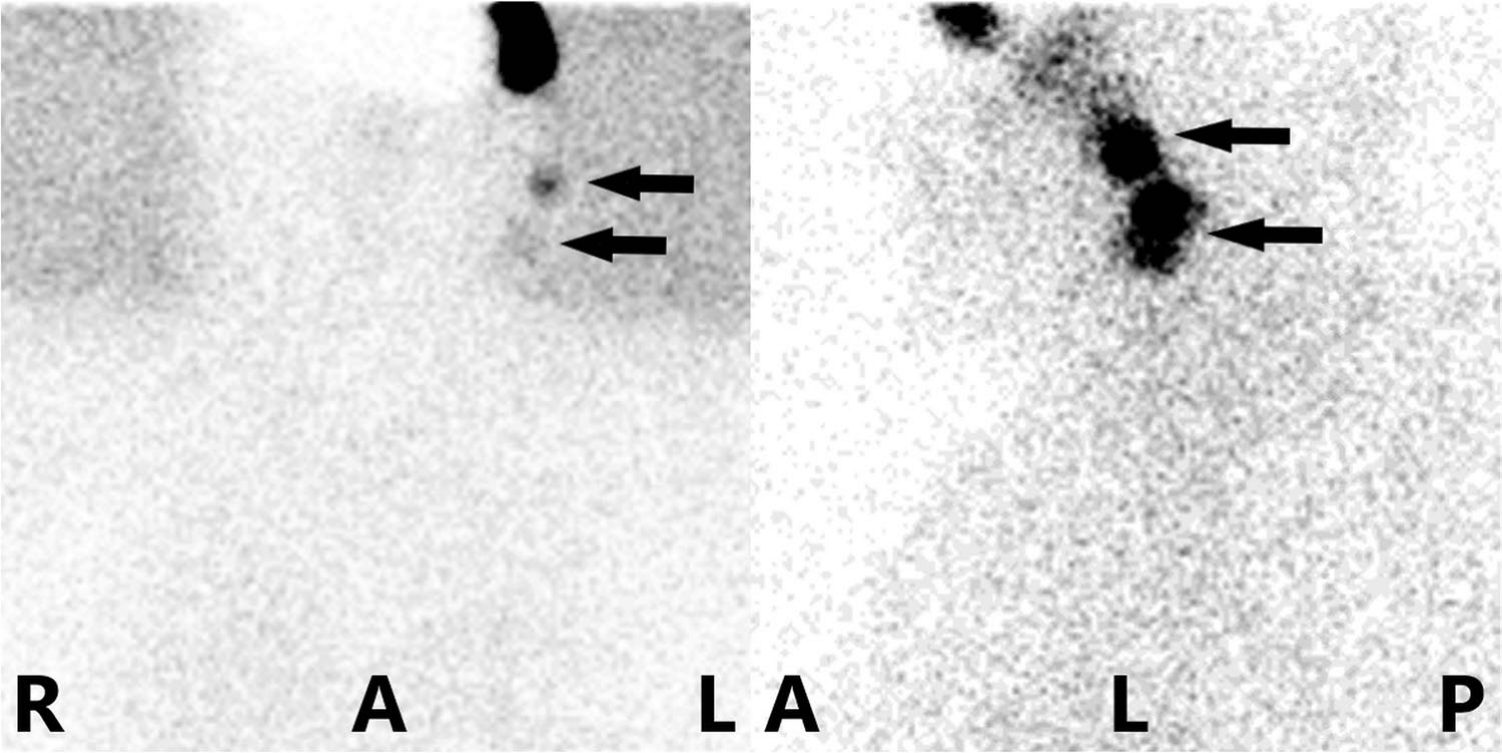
Planar lymphoscintigraphy. Planar lymphoscintigraphy in anterior/posterior projection (left) and lateral projection (right) of a patient with a superior periocular primary tumour 2 h after injection of [^99m^Tc]Tc-tilmanocept; localisation of two SLNs (arrows); abbreviations: R, right patient side; L, left patient side; A, anterior patient side; P, posterior patient side [artwork modified with Microsoft Paint, Microsoft, Redmond, USA]
